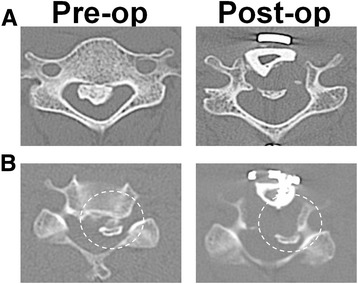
a Preoperative (*Pre-op*) and postoperative (*Post-op*) CT (computed tomography) images in the axial plane showed complete floating of ossification of the posterior longitudinal ligament (OPLL). b Insufficient floating of OPLL due to the “impingement” between OPLL and medial aspect of the uncovertebral joint
